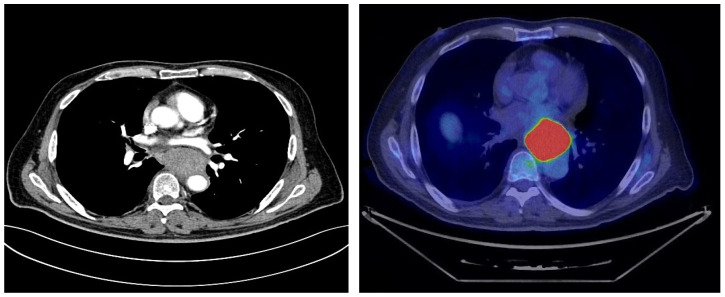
Cross-sectional CT imaging and FDG-PET demonstrated a thoracic mass in the absence of metastatic disease, but the exact site of origin remained unclear. The copyright of this figure belongs to the authors.
